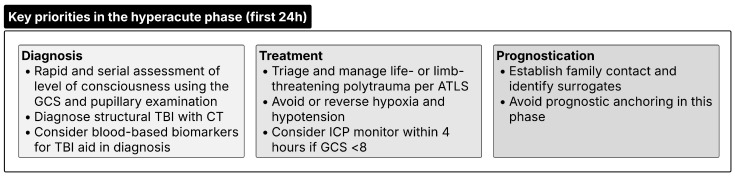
Key priorities in the hyperacute phase (first 24 h). This figure summarizes the key priorities for patients with suspected disorders of consciousness in the first 24 h after injury. GCS, Glasgow Coma Scale; TBI, traumatic brain injury; CT, computed tomography; ATLS, Advanced Trauma Life Support; ICP, intracranial pressure.
